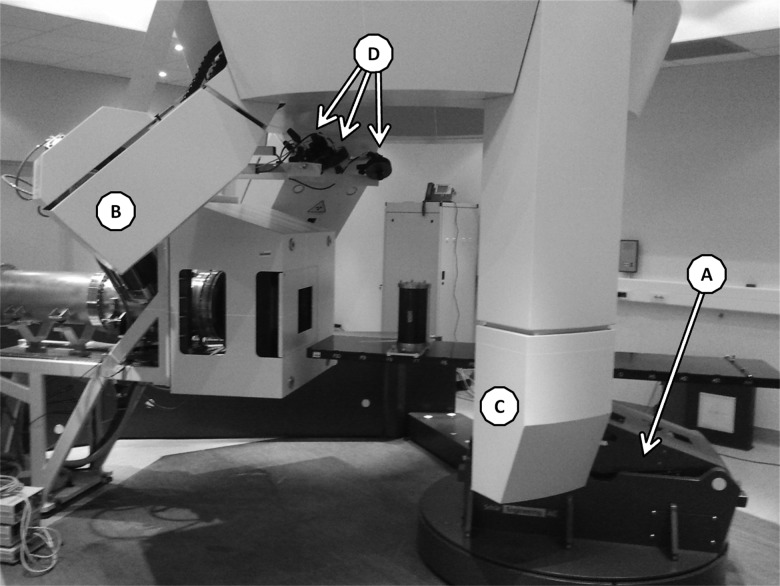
One of the three treatment rooms at CNAO. Each room is composed of three different devices. The Patient Positioning System (PPS) (A) on which is docked the couch, the Patient Verification System (PVS), an imaging system fixed to the ceiling from which kV X-ray tubes (B) and flat panels (C) are deployed, and the Optical Tracking System (OTS) (D) composed of three infrared cameras mounted on the nozzle and detecting the positions of reflecting markers fixed on the patient's contention device.
